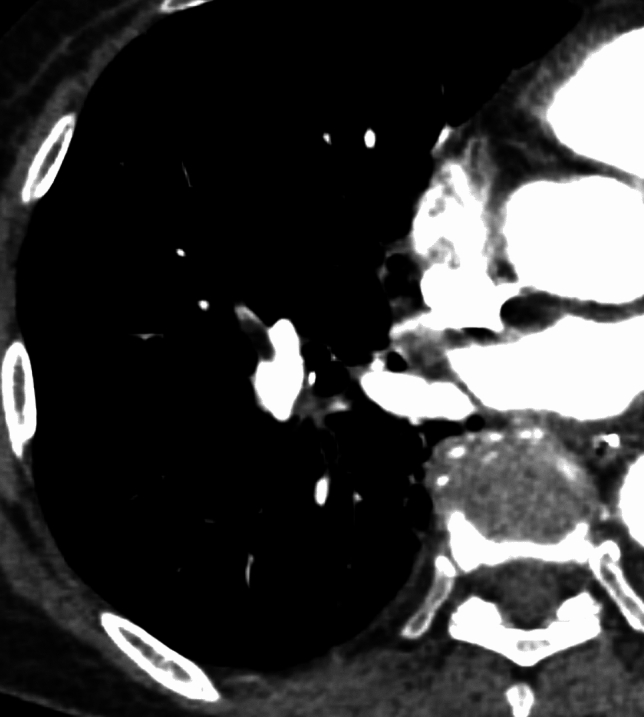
Macroscopic pulmonary fat embolism (FE). The patient was an 80-year-old woman who experienced cardiac arrest during the prosthetic replacement of the femoral stem. Contrast-enhanced CT after the return of spontaneous circulation showed multiple filling defects with a negative CT value (− 86 HU) in the pulmonary arteries
